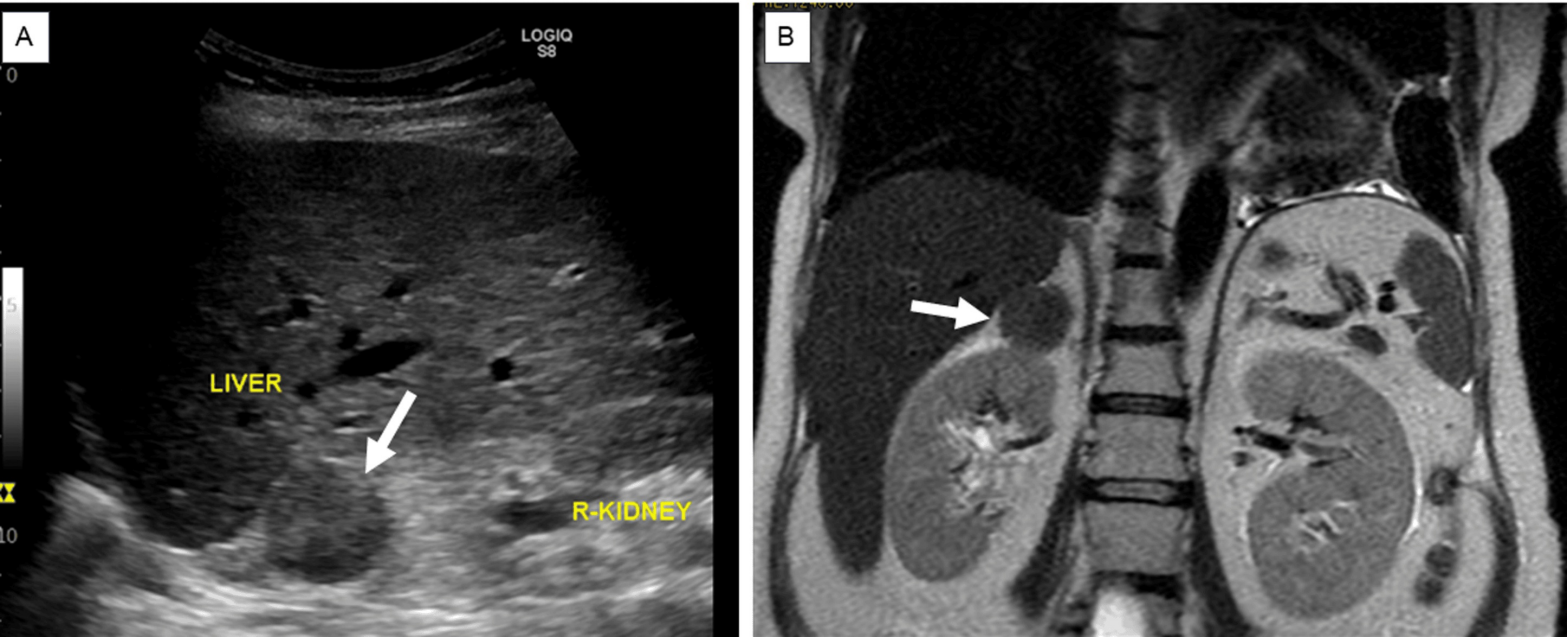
Radiological findings of the right adrenal tumor (white arrow) (A) Trans-abdominal ultrasonography image and (B) coronal section of the trunk on MRI.
